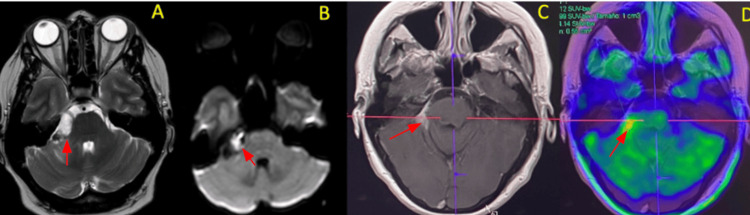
MRI and PET imaging (A, B) Initial MRI T2-weighted and DWI sequences (March 2023) revealed a right CPA lesion with heterogeneous enhancement, increased size, and extension into the internal auditory canal. (C, D) Contrast-enhanced MRI and ¹⁸F-FDG brain PET demonstrated focal hypermetabolism in the right CPA region, with a maximum standardized uptake value of 10.3. CPA, cerebellopontine angle; DWI, diffusion-weighted imaging
